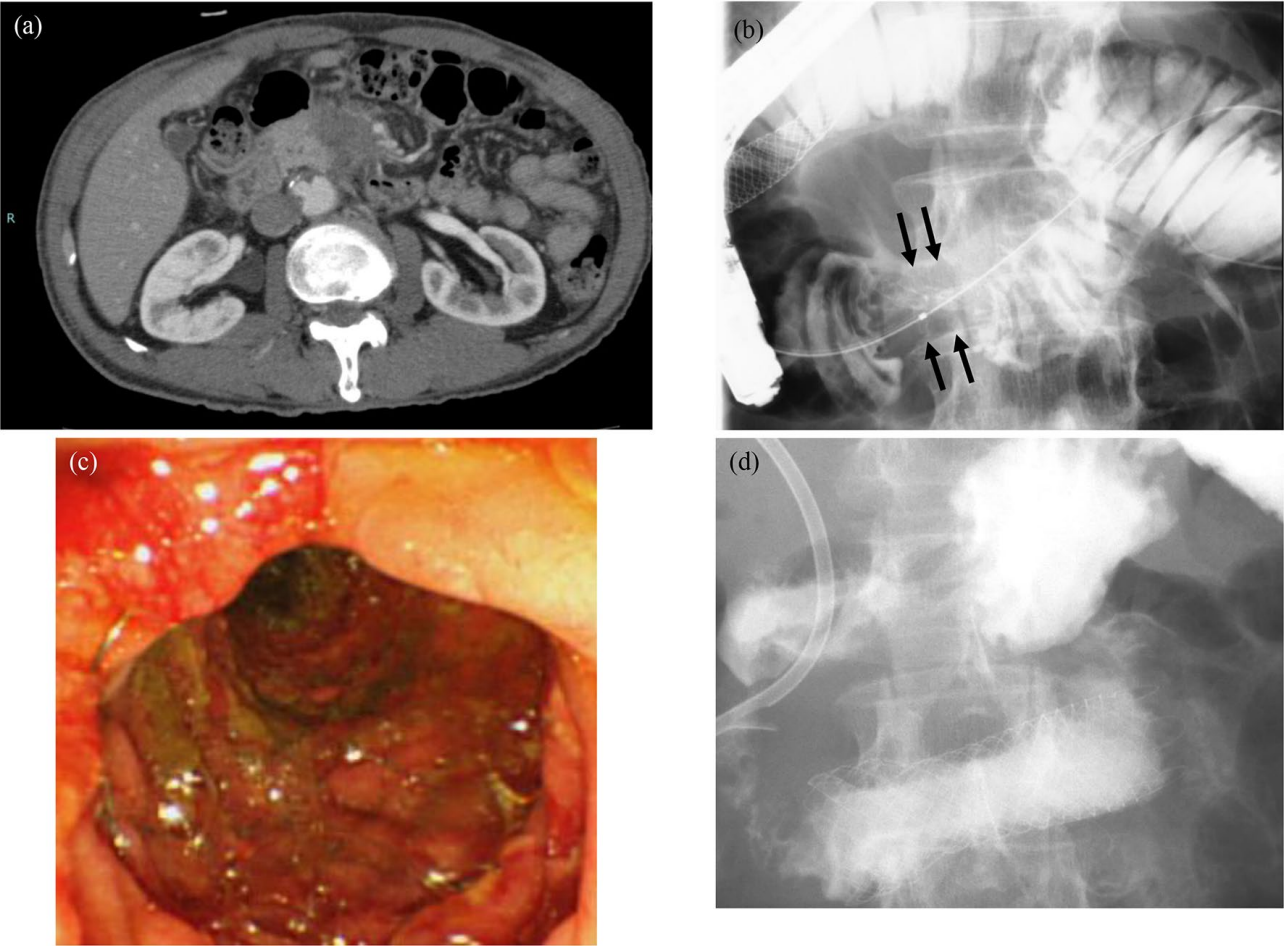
Images of a 70-year-old man with metastatic pancreatic cancer. Both his Gastric Outlet Obstruction Scoring System (GOOSS) and Glasgow Prognostic Score were 0 before duodenal stent (DS) placement. (a) Computed tomography revealed a pancreatic tumor with duodenal invasion. (b) Fluoroscopic imaging shows a severe D3 stricture (arrows). Endoscopic (c) and fluoroscopic (d) images show the resolution of the stricture after DS placement, which has improved the GOOSS to 3. He received chemotherapy and achieved long-term survival (901 days) without re-intervention of the DS.
